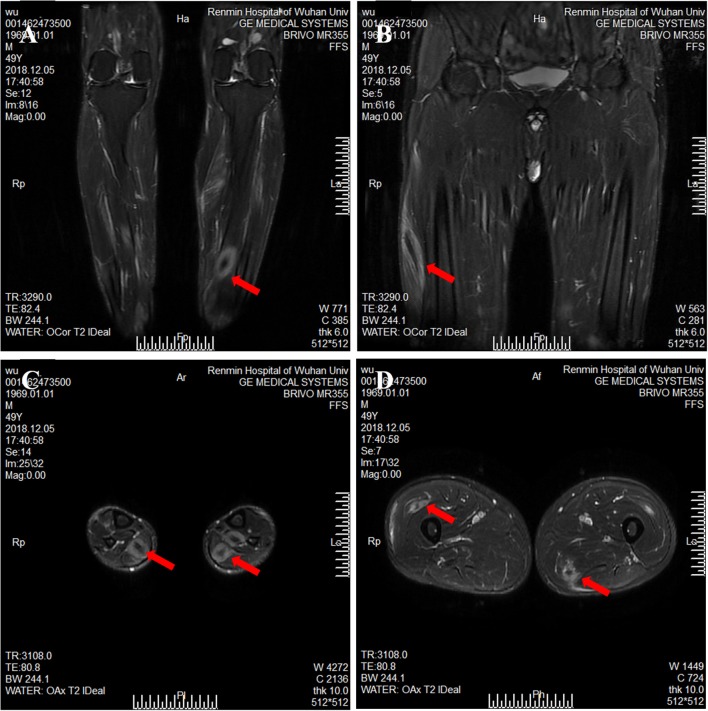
Magnetic resonance imaging of calf and thigh masses. (A) A coronal T2-weighted image shows a mass with a clear boundary, with a long T2 signal and a short T2 signal at the center of the mass in the calf. (B) A coronal T2-weighted image shows muscular enlargement with increasing signal intensity over the muscles and subcutaneous areas in the thigh. (C) The axial T2-weighted image showed a clear boundary mass with a long T2 signal and a short or equal T2 signal at the center of mass in the gastrocnemius and soleus muscle. (D) The axial T2-weighted image shows a mass with a blurry boundary, with a long T2 signal and a short T2 signal at the center of mass in the lateral femoral muscle and adductor muscle.
